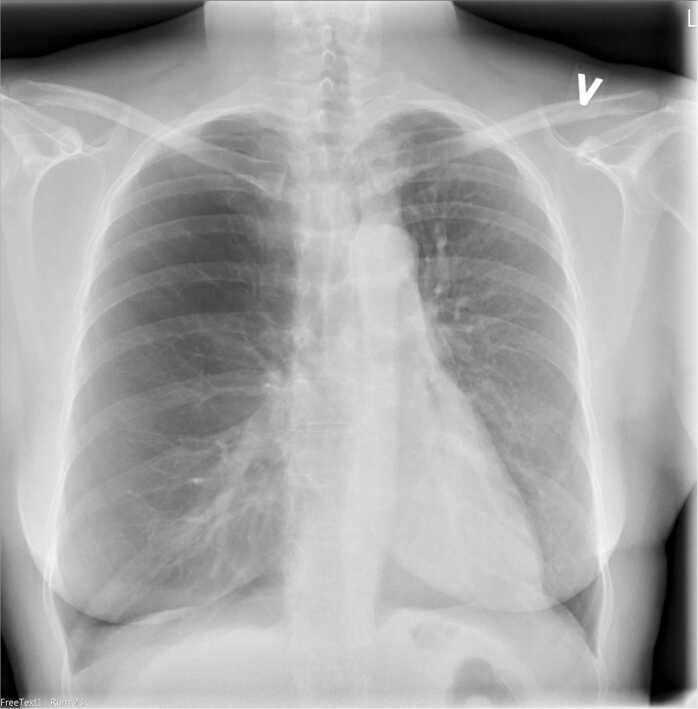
Chest X-ray before LVRC treatment. LVRC, lung volume reduction coils.
